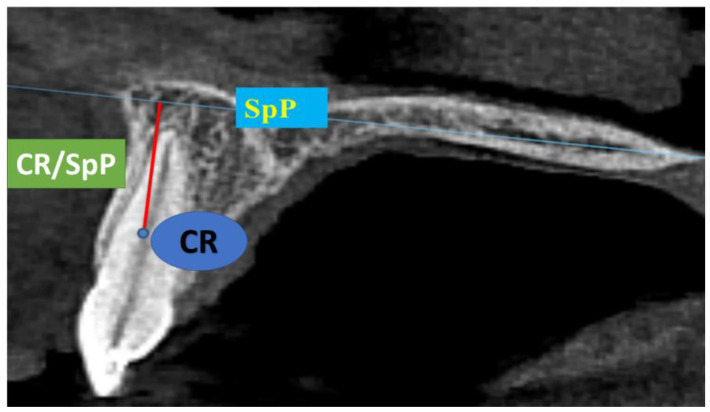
Maxillary plane (SpP), tooth center of resistance (CR), and the distance from SpP to CR on a CBCT sagittal projection.
